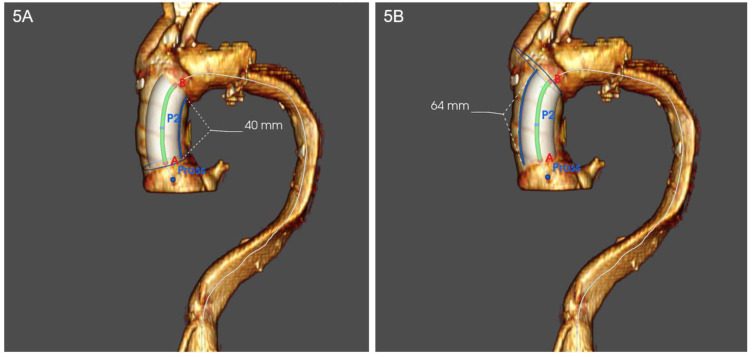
(A,B) Representation of inner and outer curves of ascending aorta in volume rendering 3D computed tomography angiogram. Inner curve (A) appears shorter than outer curve (B).
